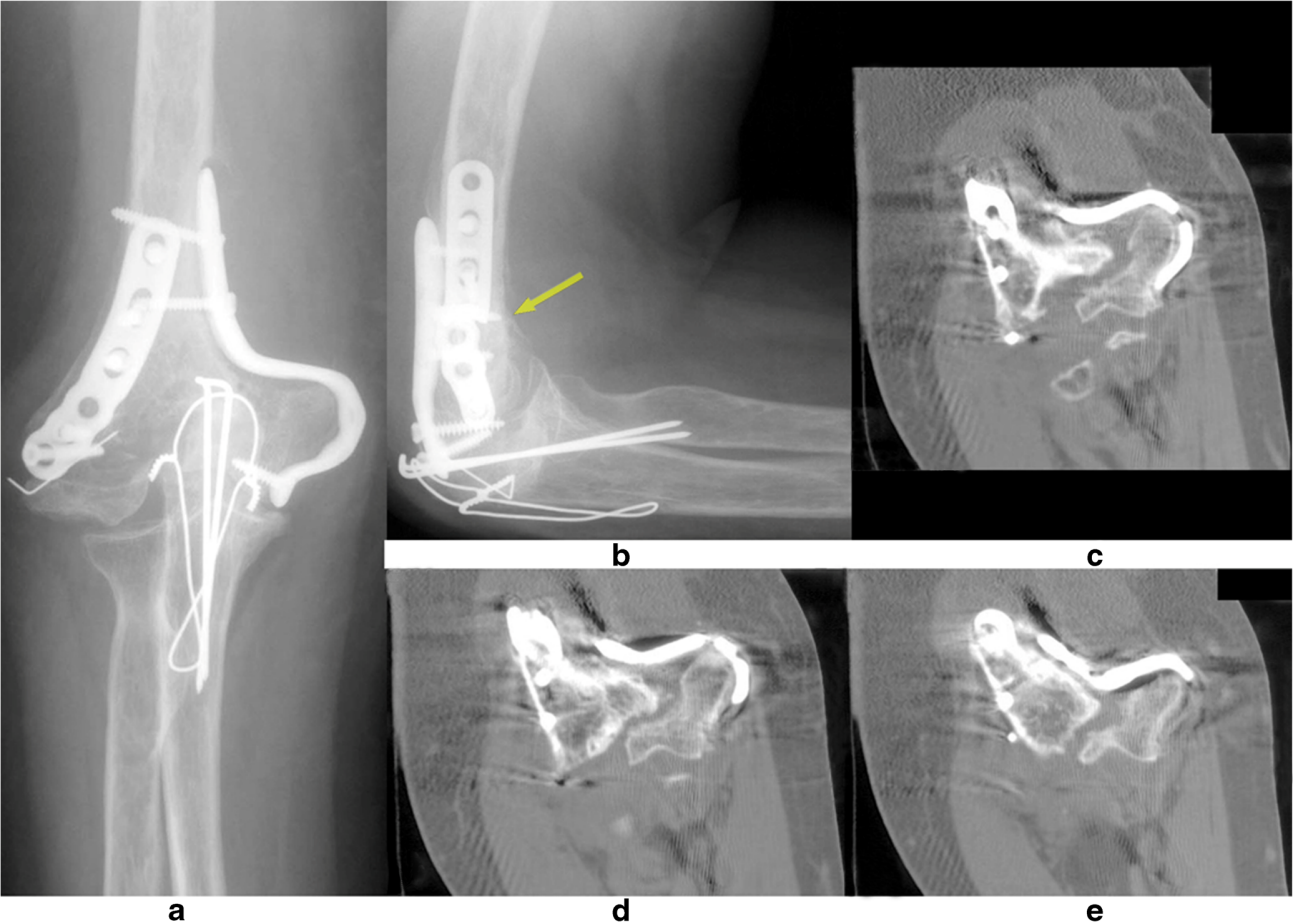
A 60-year-old female presents 8 months following ORIF of a right-sided distal humeral fracture with complaints of pain at the fracture site and limited range of motion of the elbow. Radiographs (a, b) and CT scan images (c–e) reveal a nonunion, and loss of fixation with plate breakage (medial side).
